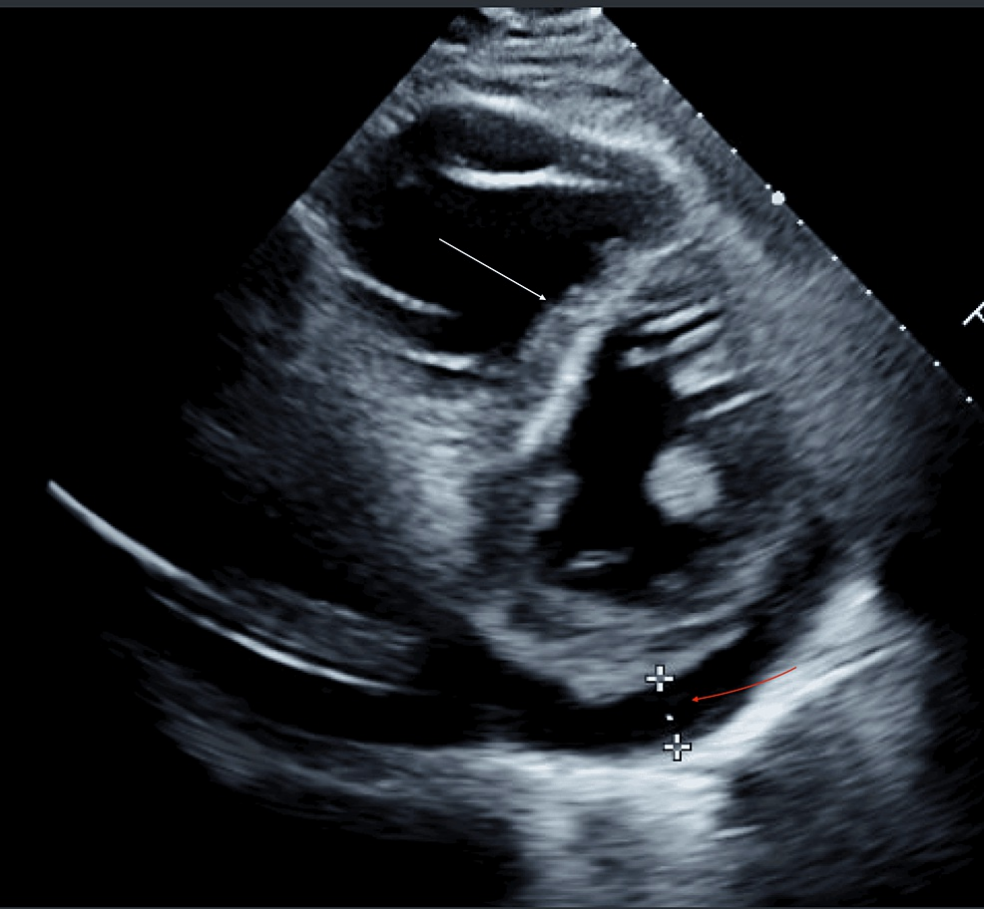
Short-axis transthoracic echocardiogram post-pericardiocentesis The image shows an enlarged RV with septal flattening (white arrow) consistent with RV strain not seen on the initial echocardiogram. There is also mild posterior pericardial effusion (red arrow). Interval resolution of the anterior pericardial effusion is seen RV: right ventricle
